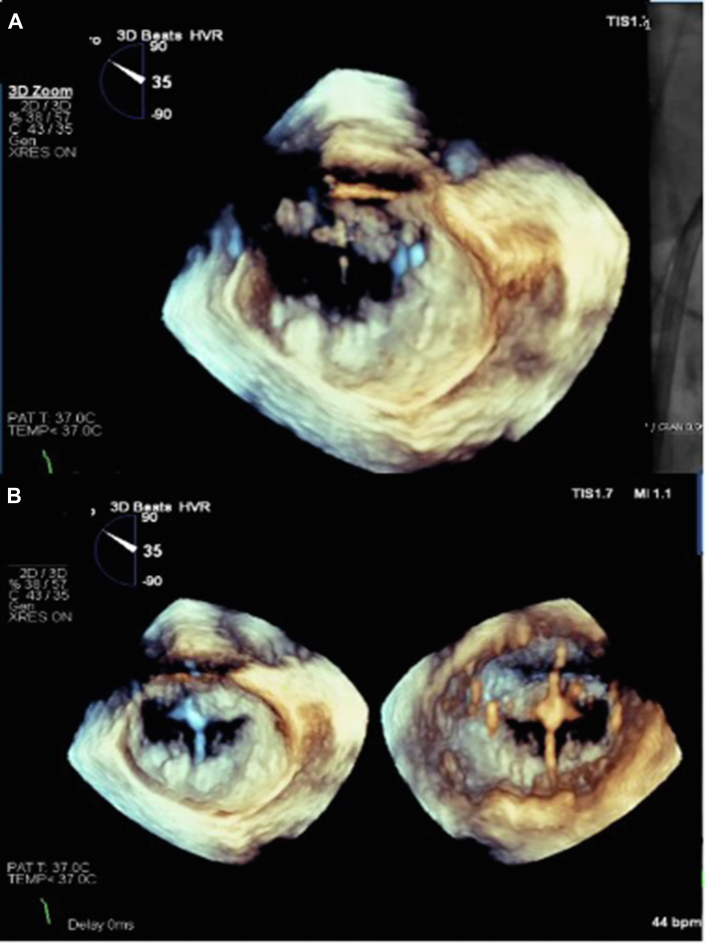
Mitral Valve Intracardiac Echocardiography Four-dimensional intracardiac echocardiographic view of the mitral valve from the left atrium (A) before and (B) after wire placement.
